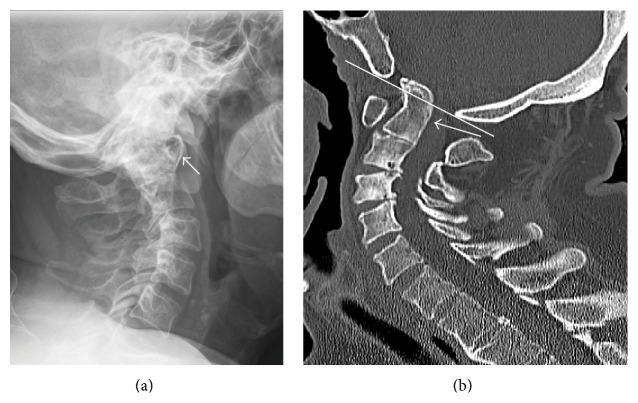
Lateral radiograph of patient with severe cranial settling. Of note, the settling is so severe that the dens is not identifiable due to overlying mastoid air cells and skull base,* arrow* identifying the anterior arch of C1 (a). Sagittal reconstructions of computed tomography of the cervical spine in the same patient. Note the anterior arch of C1 is at Station III and the dens (*arrow*) projects through the inferior margin (*line*) of the foramen magnum (b).
